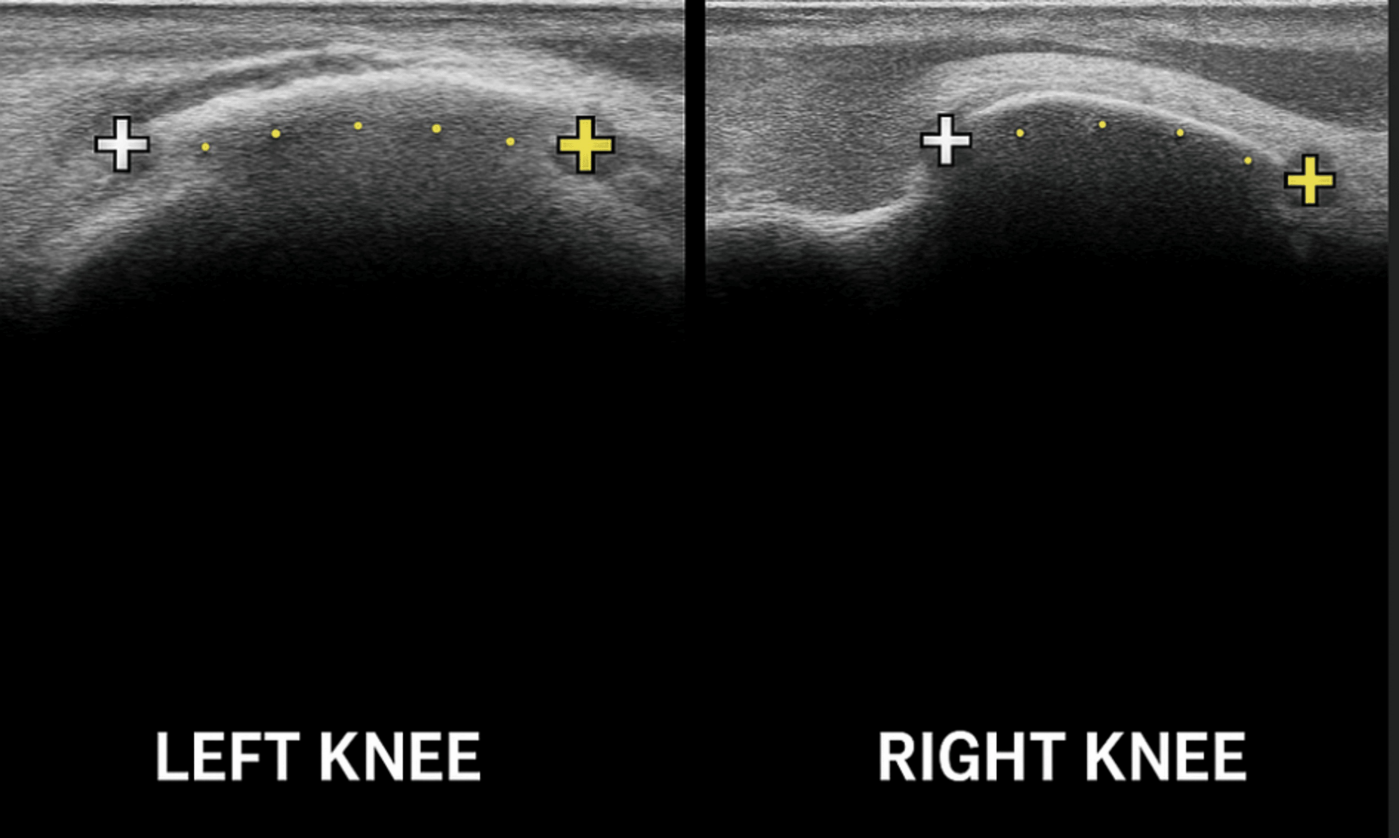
Ultrasound Image of both knees confirming Pellegrini-Stieda calcification
